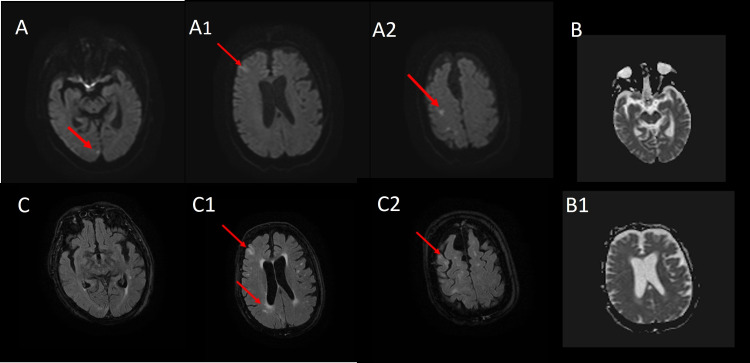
MRI acquired subsequently shows multiple right occipital, frontal, and parietal cortical lesions characterized by the high signal in DW images (A, A1, A2) and FLAIR (C, C1, C2), but without corresponding lesions on the ADC map (B, B1). FLAIR: fluid‐attenuated inversion recovery; DW: diffusion-weighted; ADC: apparent diffusion coefficient
